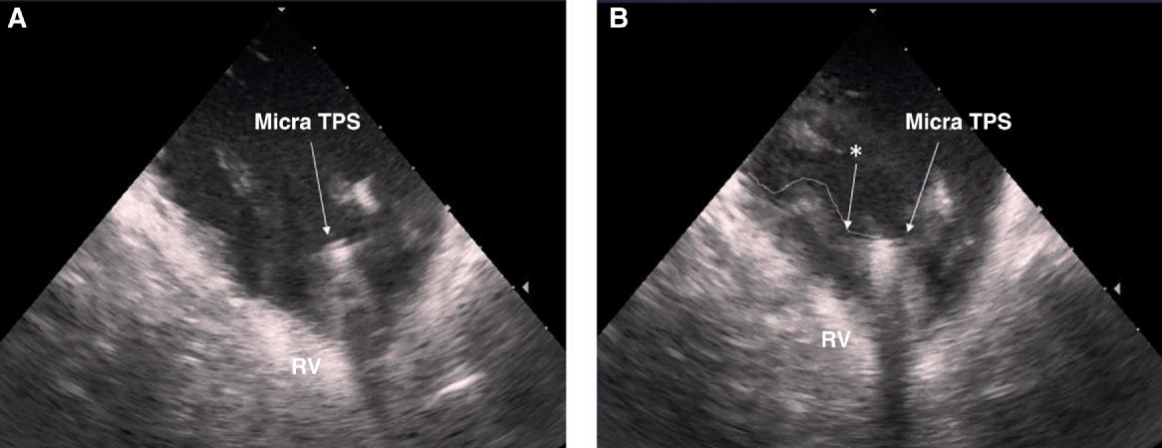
Intracardiac echocardiography. (*A*) Intracardiac echocardiography in a case of successful retrieval of the Micra TPS. The proximal retrieval feature is visible, and the TPS was not covered by surrounding tissue. (*B*) Intracardiac echocardiography in a case of unsuccessful retrieval. Surrounding tissue was severely adherent to the TPS, which was partially embedded within the cardiac endocardium (*denoted by the asterisk). RV, right ventricle; TPS, transcatheter pacing system.
